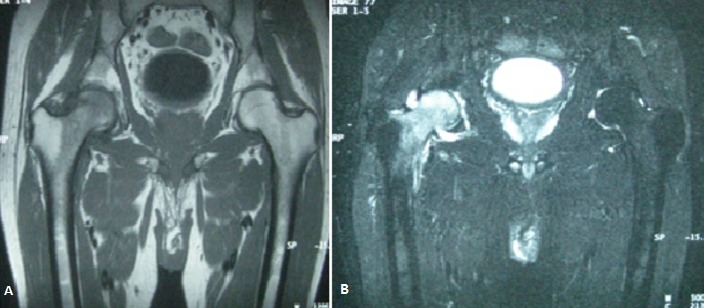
(A) MRI of the hips: diffused medullary edema of the neck and right femoral head in hyposignal T1 and hypersignal STIR with discreet intra-articular effusion blade; (B) suggestive of right hip algodystrophy
